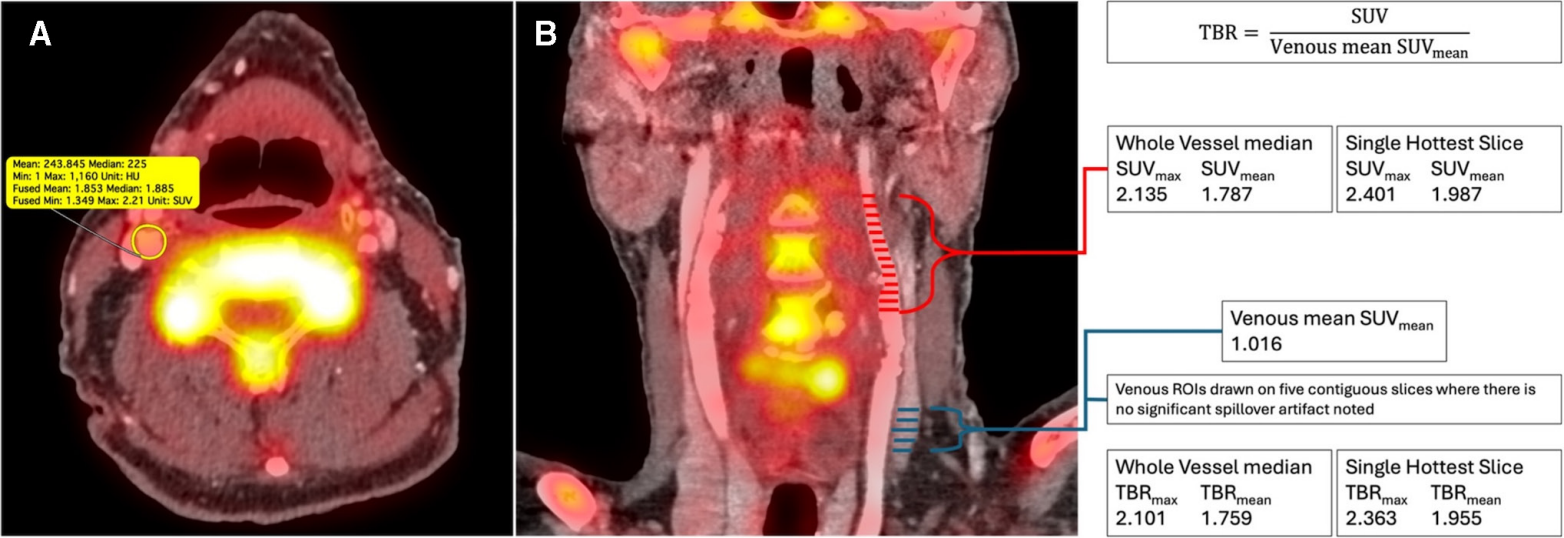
Evaluating tracer uptake. A, Axial positron emission tomography (PET)/computed tomography angiography (CTA) with an example region of interest (ROI), showing standardized uptake value (SUV) data. B, coronal PET/CTA with arterial ROIs displayed around the carotid bifurcation (red lines), and ROIs in a proximal vein unaffected by spillover from adjacent structures (blue lines). Target-to-background ratio (TBR) statistics are calculated by taking the relevant arterial SUV statistic and dividing by the mean of the SUV_mean_ obtained from the venous ROIs.
